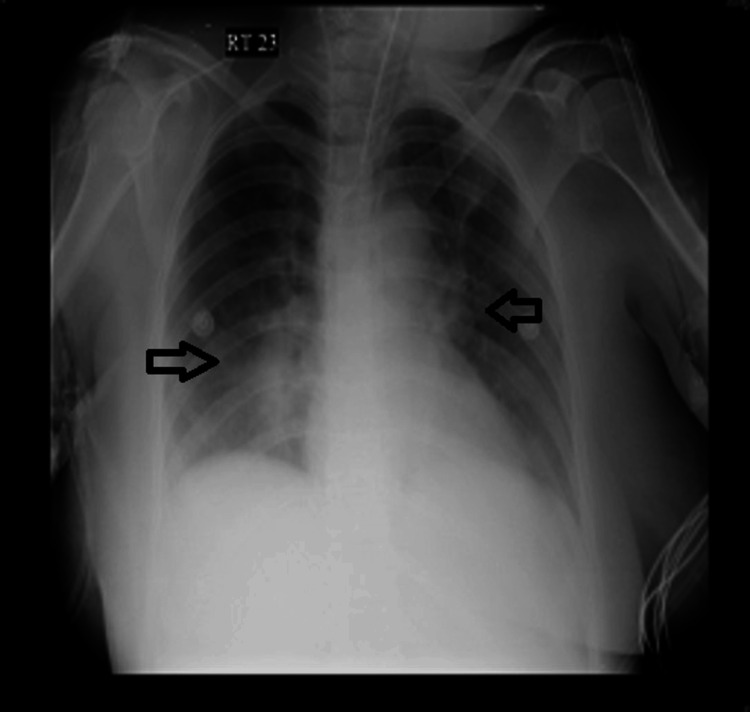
Chest radiograph done after intubation showing bilateral hilar prominence
